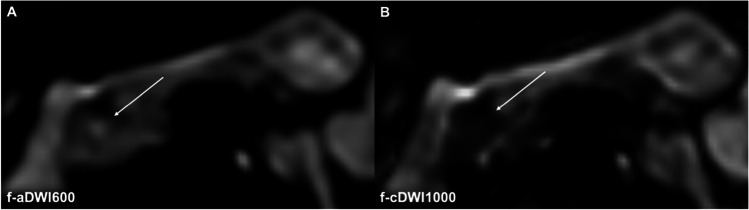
73-year-old women with a benign IPMN. Malignant IPMN was misdiagnosed based on the standard DWI at *b* = 600 s/mm^2^ (A) but correctly diagnosed as benign reviewing the computed high-*b*-value DWI at *b* = 1000 s/mm.^2^ (B)
